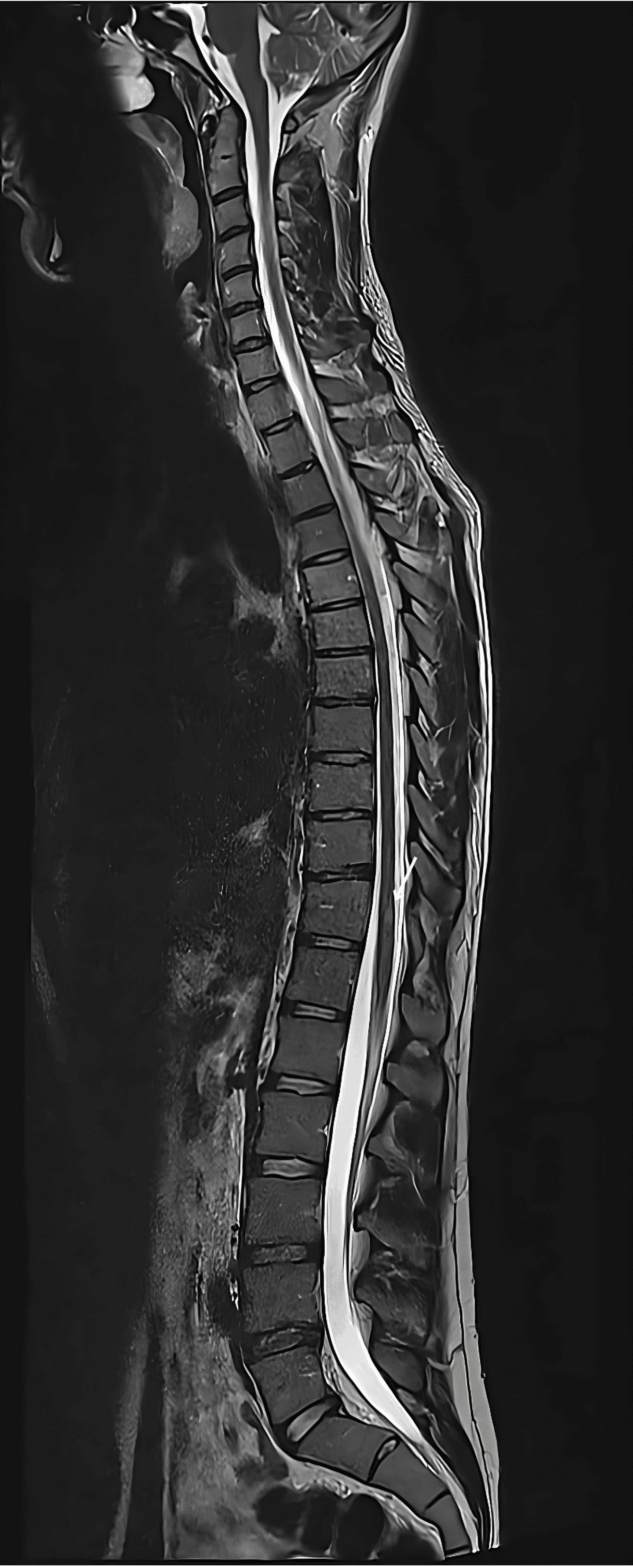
Spinal MRI, sagittal T2-weighted sequence. White arrow highlights focal hyperintense lesion at T11
